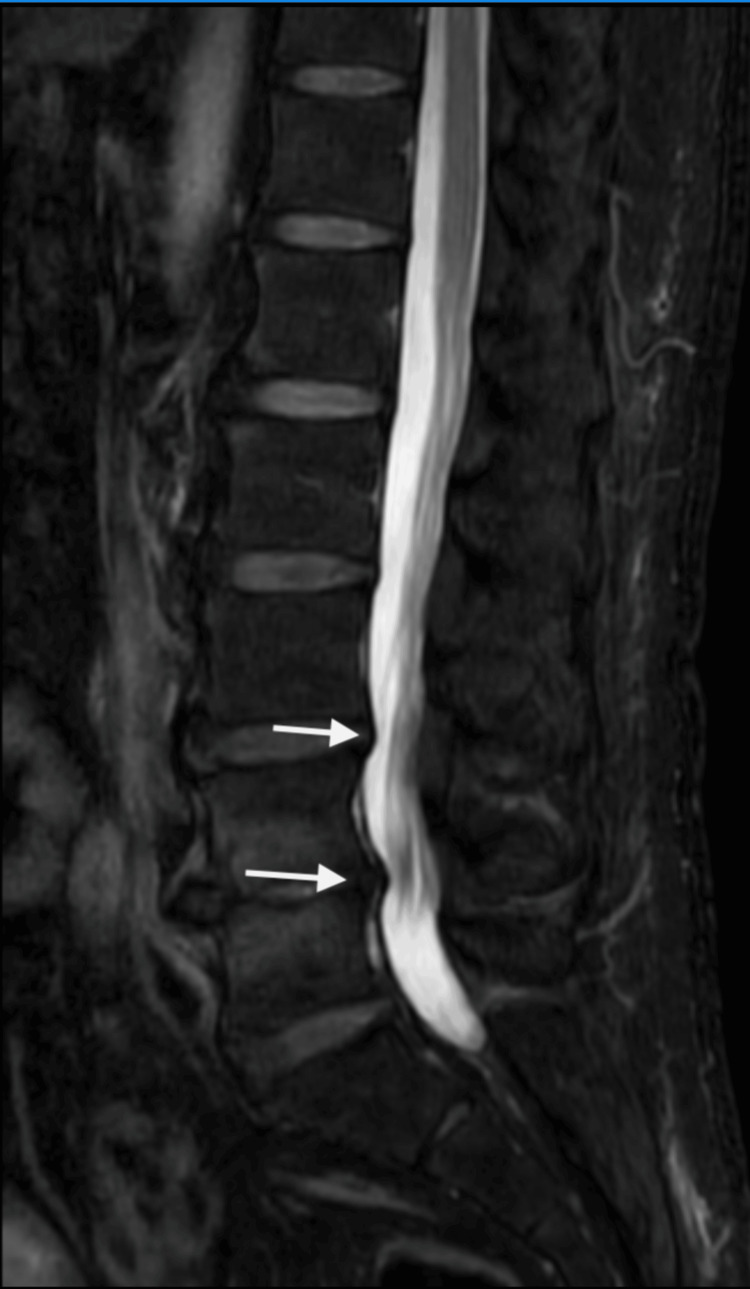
Lumbar spine MRI MRI of the lumbar spine showing moderate spinal stenosis at L3-L4 and L4-L5 (arrows). MRI: magnetic resonance imaging
